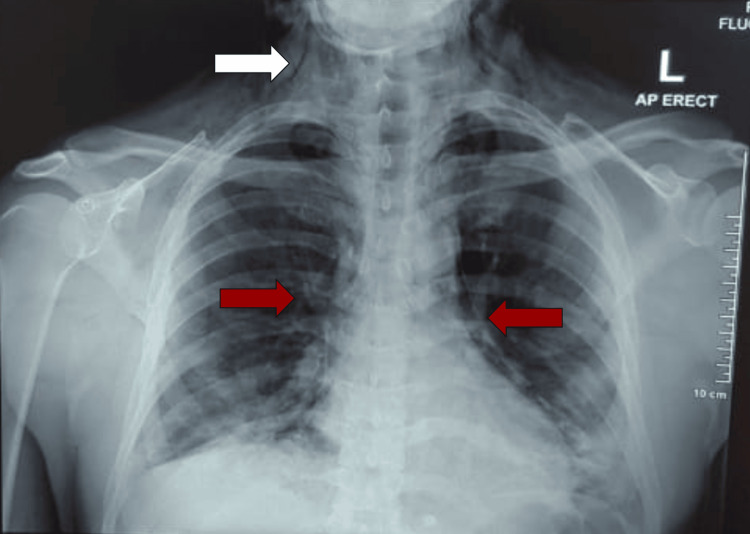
Anterior-posterior chest radiograph showing the curvilinear radiolucent streaks of mediastinal margins (red arrows) and subcutaneous air in the neck (white arrow).
